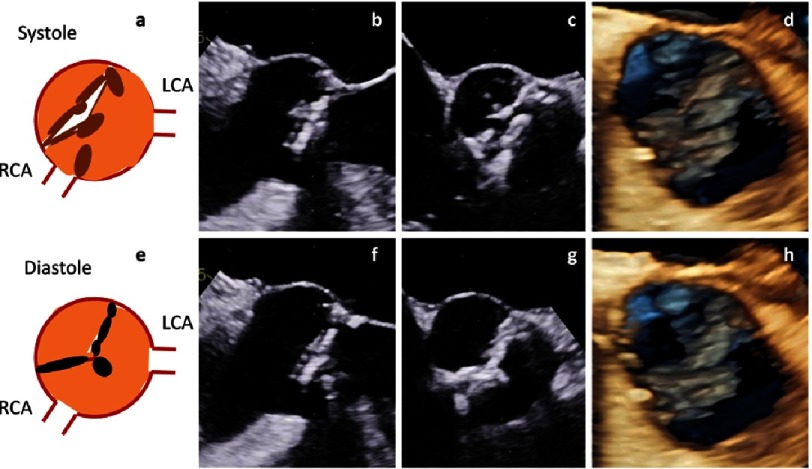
Calcification and retraction of the cusps in a patient with a true bicuspid AV. Scheme of the short axis view (a), 2D transesophageal long axis view (b), 2D transesophageal short axis view (c) and 3D transesophageal en-face view of the AV (d) are shown above during systole. Corresponding views are shown below during diastole (e-h).
